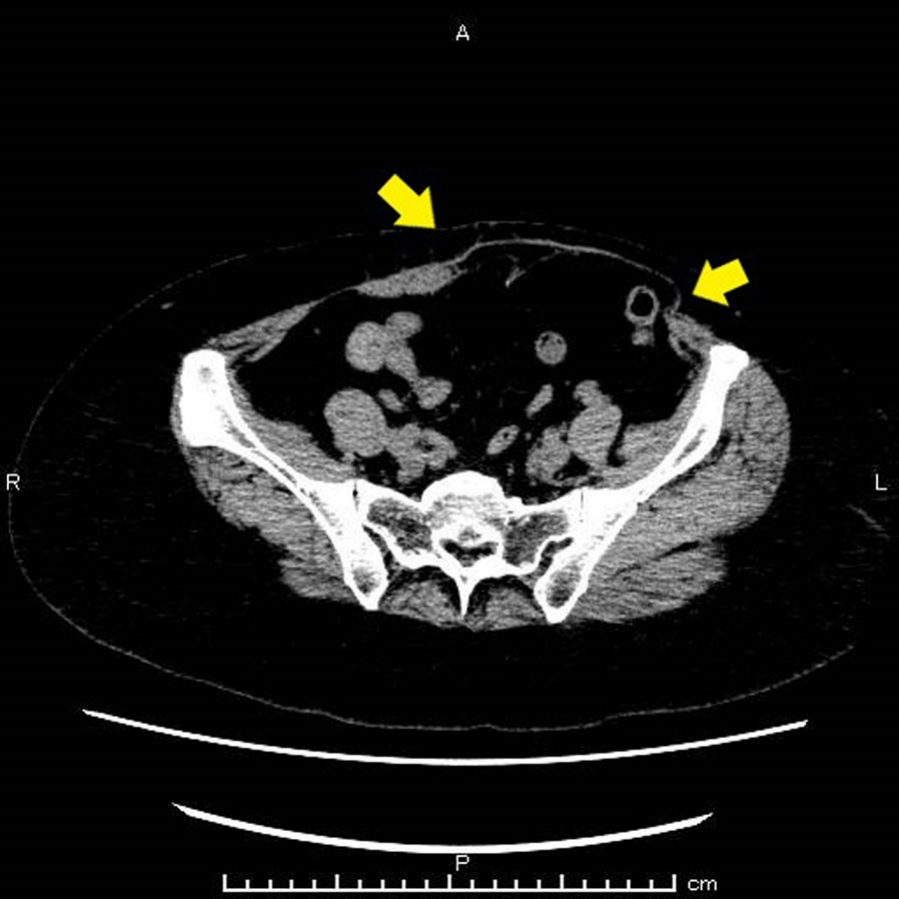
Abdominal computed tomography. Abdominal computed tomography showing a left lower abdominal bulge attributed to atrophy of the left rectus abdominis muscle with no evidence of a fascial defect (yellow arrow)
